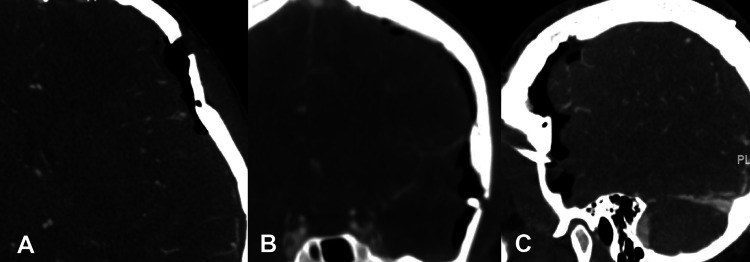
Post-operative CT angiogram images. A: Axial; B: Coronal; and C: Sagittal images, demonstrating no evidence of the bridging vessels seen in pre-operative imaging.
